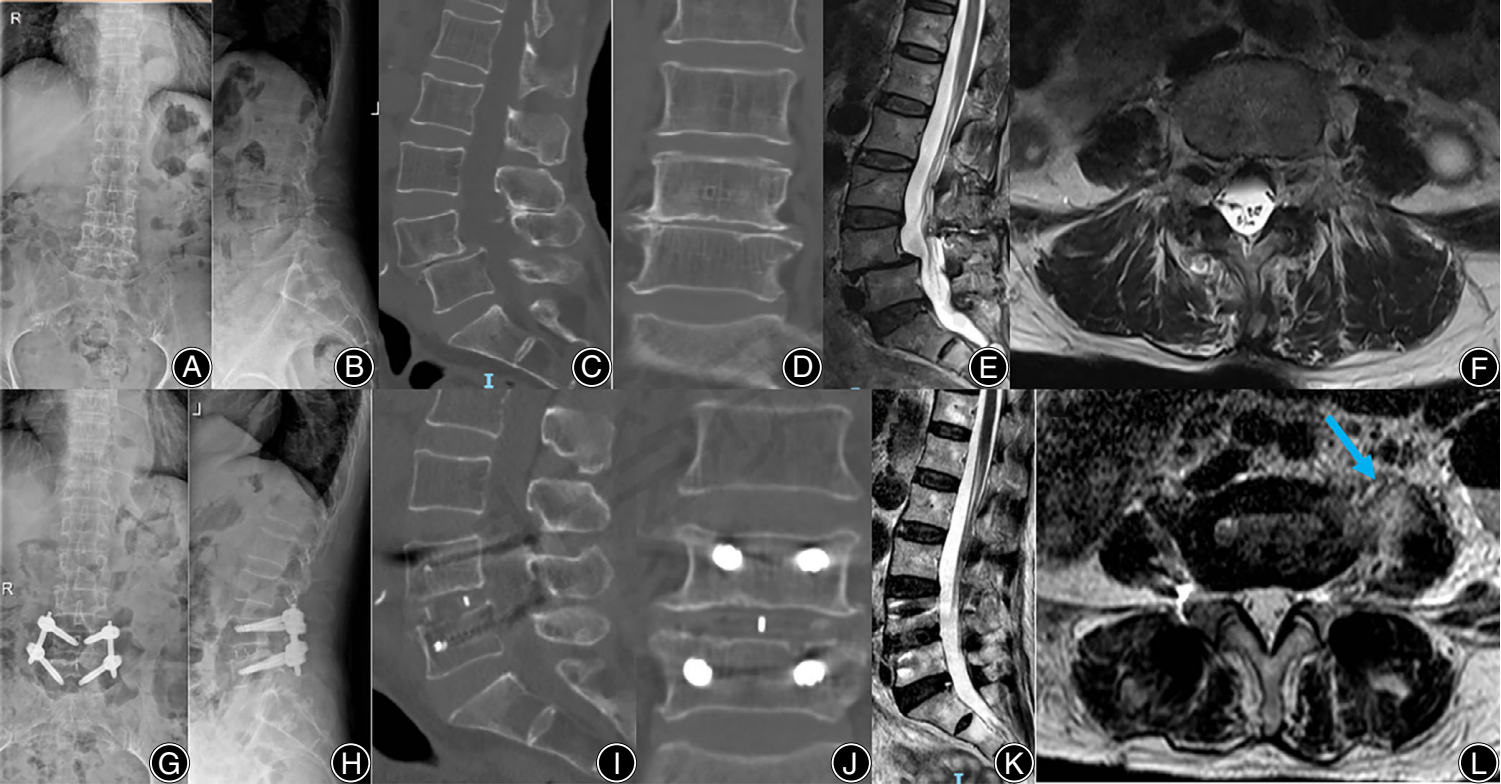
Typical case. A 72‐year‐old woman, presented with pain and numbness in both lower extremities for 6 years and worsened for 2 weeks. (A, B) Preoperative lumbar X‐ray; (C, D) Preoperative lumbar CT; (E, F) Preoperative lumbar MRI. Preoperative images showed the L4 spondylolisthesis and stenosis at L4‐5; (G, H) Postoperative lumbar X‐ray showed L4 was reduced; (I, J) Postoperative lumbar CT showed distraction of disc height; (K, L) Postoperative lumbar MRI showed satisfactory decompression and a huge swelling of the left psoas major (arrow).
